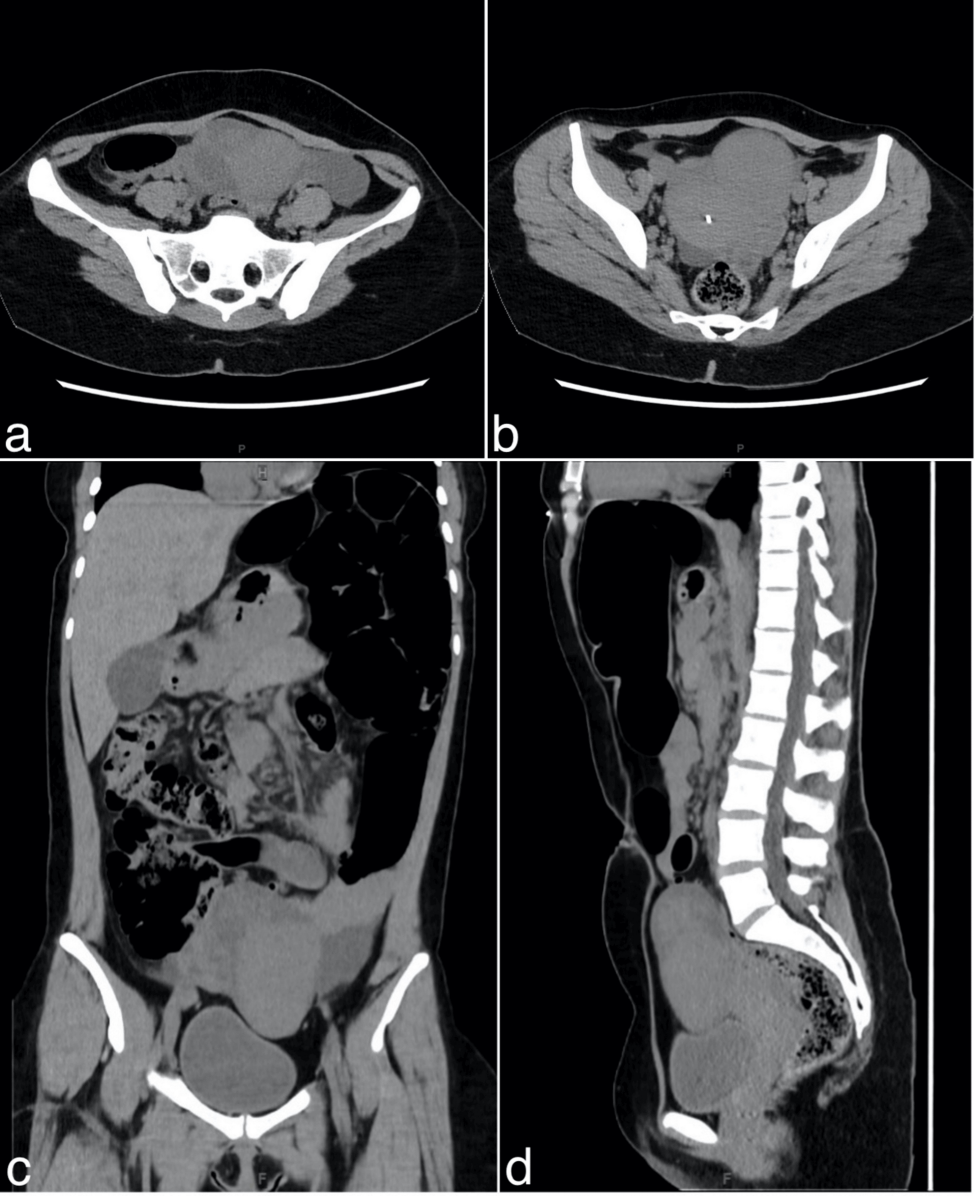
CT abdomen, low dose without IV contrast (Revolution CT, GE Healthcare®) Axial (a, b), coronal (c), and sagittal (d) CT images show a soft tissue mass anterior, cranial, and just to the left of the uterus with surrounding free abdominal fluid. CT: computed tomography, IV: intravenous
